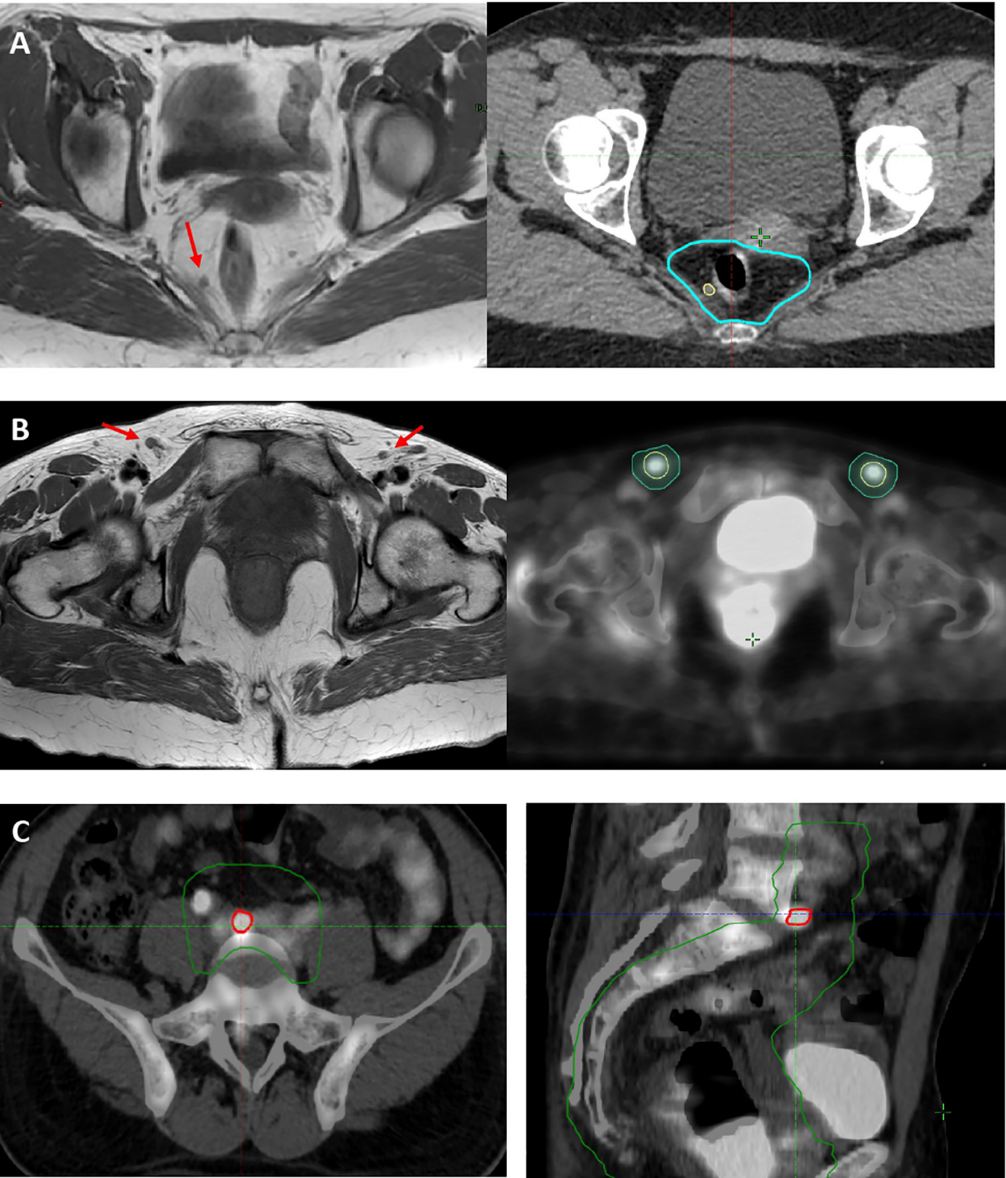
(A) Case of mesorectal PET−/MRI+ LN (red arrow), included as mesorectal GTV N (yellow line) in 54 Gy high-dose volume (light blue line). (B) Case of disease upstage and dose escalation on MRI− (red arrows)/PET+ (yellow line) inguinal LN. (C) Case of disease upstage with a dose escalation on MRI−/PET+ common iliac LN (red line) and inclusion of common iliac LN level to 45 Gy low-dose volume (green line).
